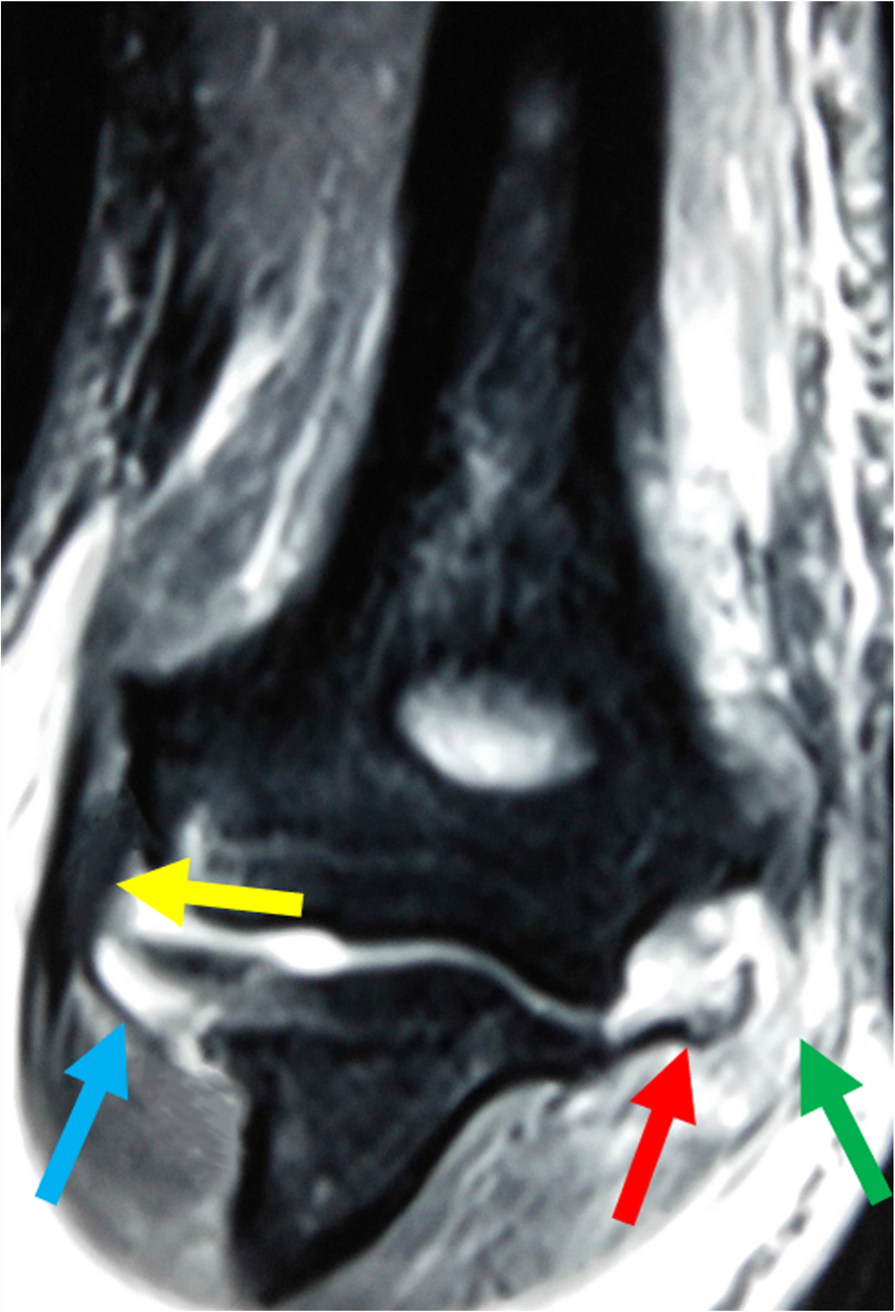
T2-weighted coronal image from magnetic resonance imaging. The medial collateral ligament (red arrow) is avulsed from its humeral footprint. The flexor-pronator tendon is torn as well (green arrow). There is an increased signal in their origin on the medial epicondyle. The lateral collateral ligament (yellow arrow) and the lateral ulnar collateral ligament (blue arrow) are intact
